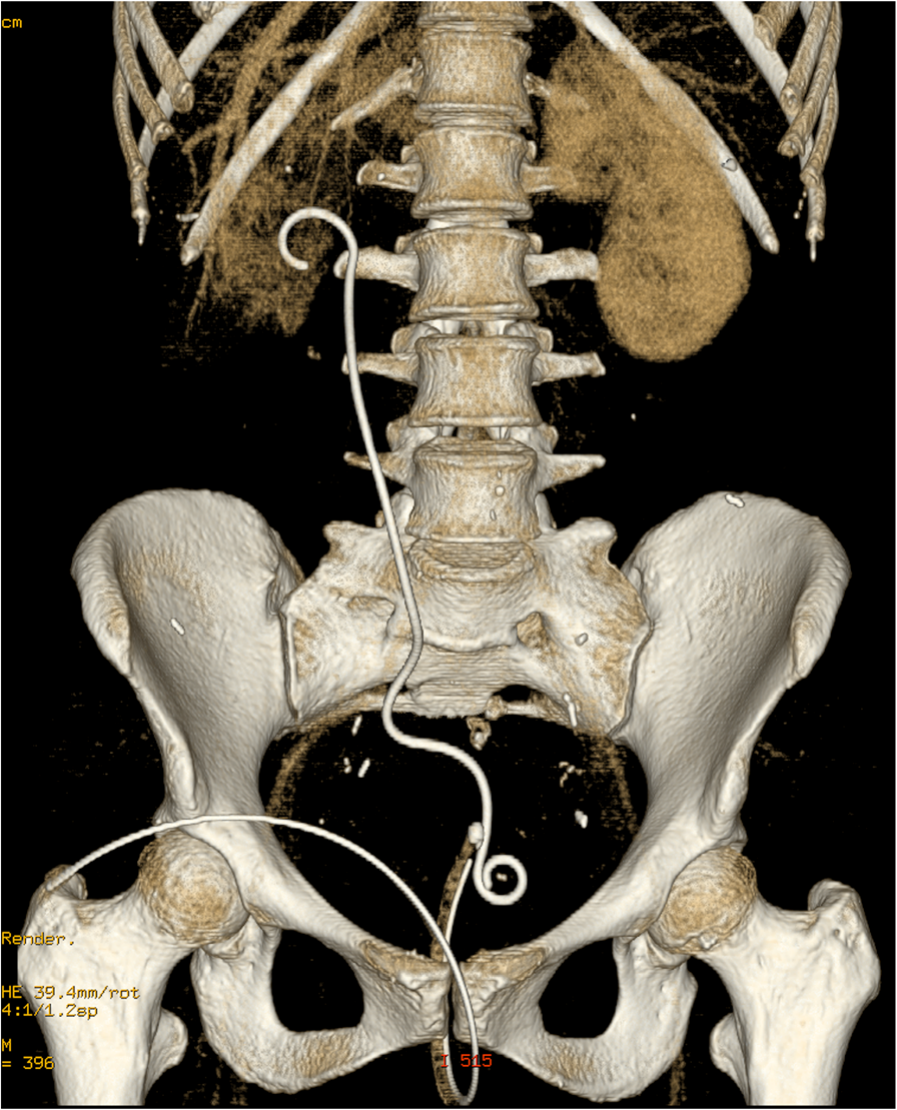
3D rendering of the CT scan showing a cross-over course of the right ureteral stent at the level of mid-sacrum, while the stent distal coil is placed on the left side of the midline, defined by the presence of the bladder catheter
